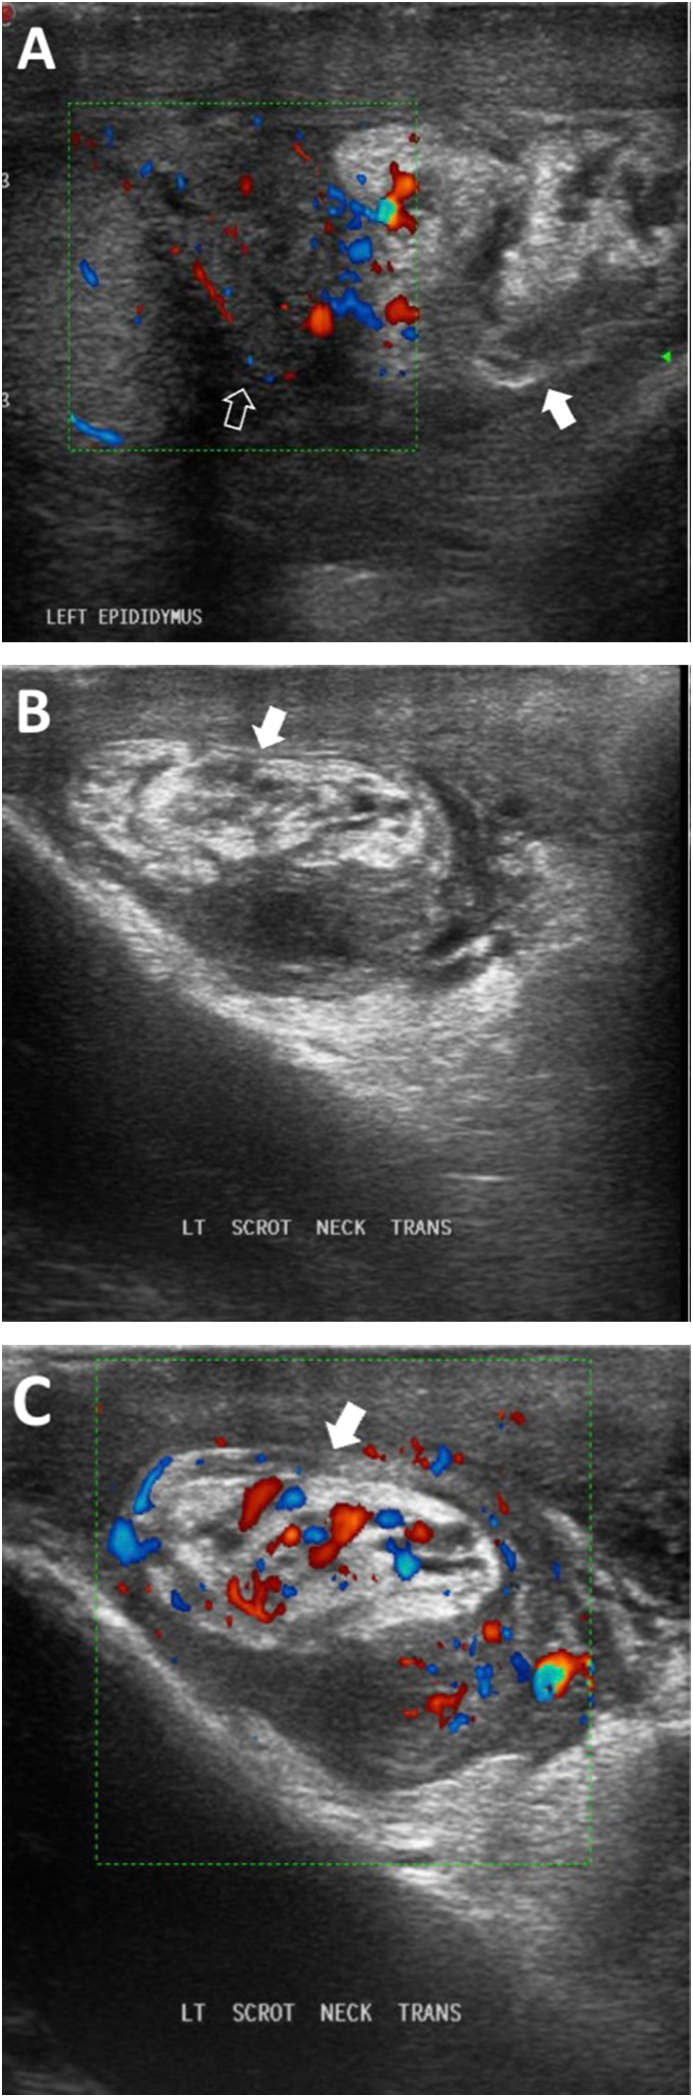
(A,B , C) Gray scale and color Doppler US images showing heterogenous echogenicity lesion (solid arrow) adjacent to the head of left epididymis (empty arrow) with increased vascularity of both. (For interpretation of the references to color in this figure legend, the reader is referred to the Web version of this article.)
